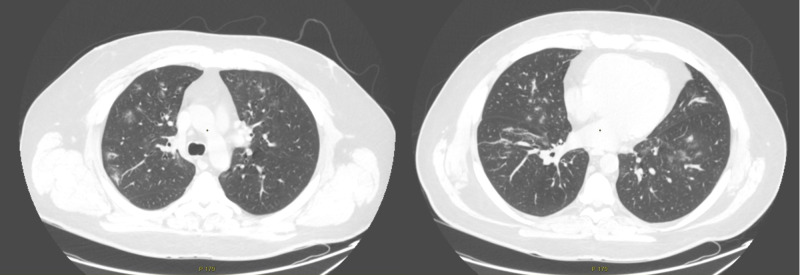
Axial chest CT scans CT scans were taken on the eighth day of the disease onset and revealed up to 45% damage to both lungs with the progression of ground-glass opacities to infiltration CT: computed tomography
